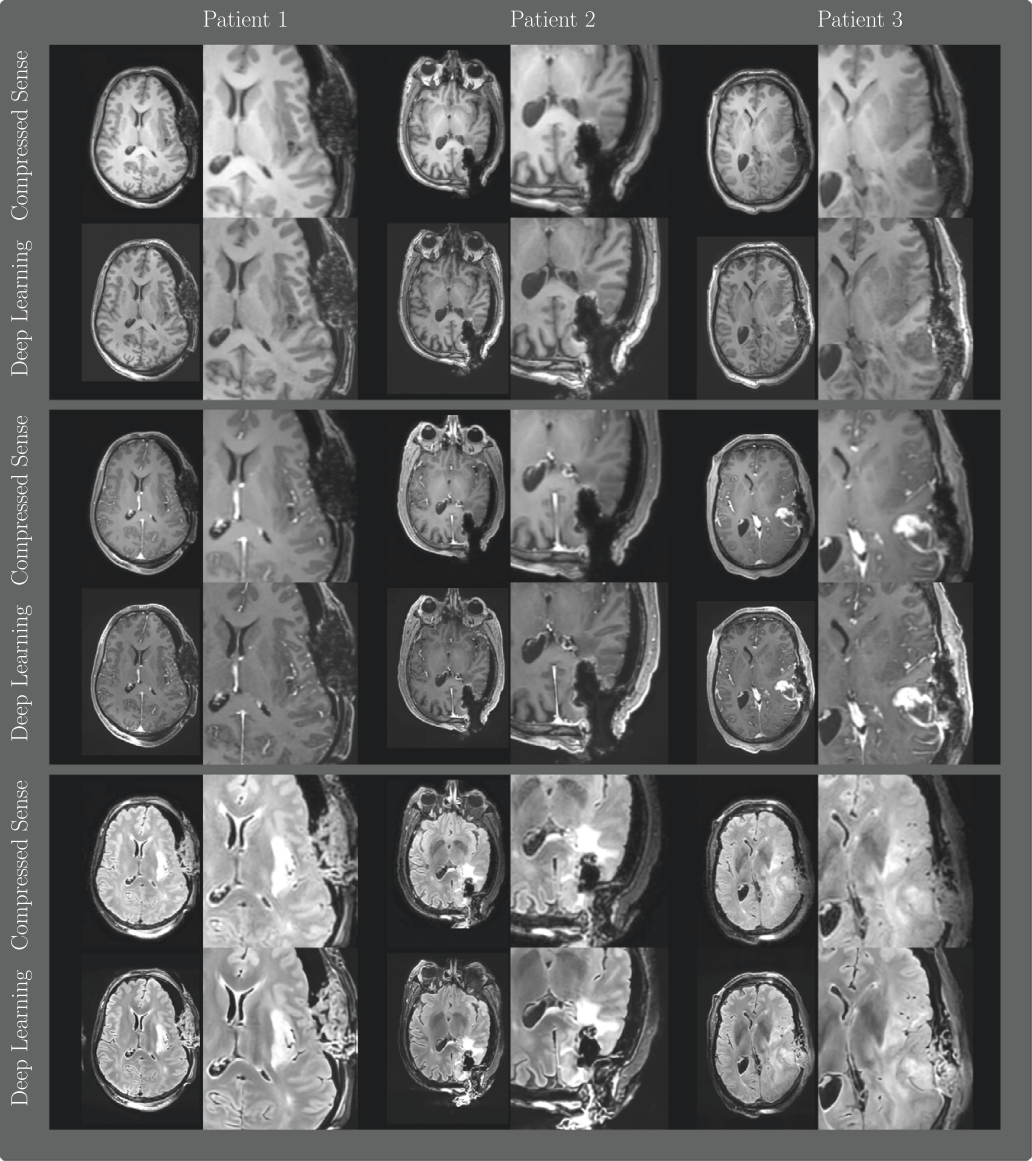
Representative examples of the DL and CS reconstructions from four different patients, depicting T1-weighted pre-contrast, T1-weighted post-contrast, and T2-weighted FLAIR scans. Window level was chosen between 0.05 and 0.995 percentiles
